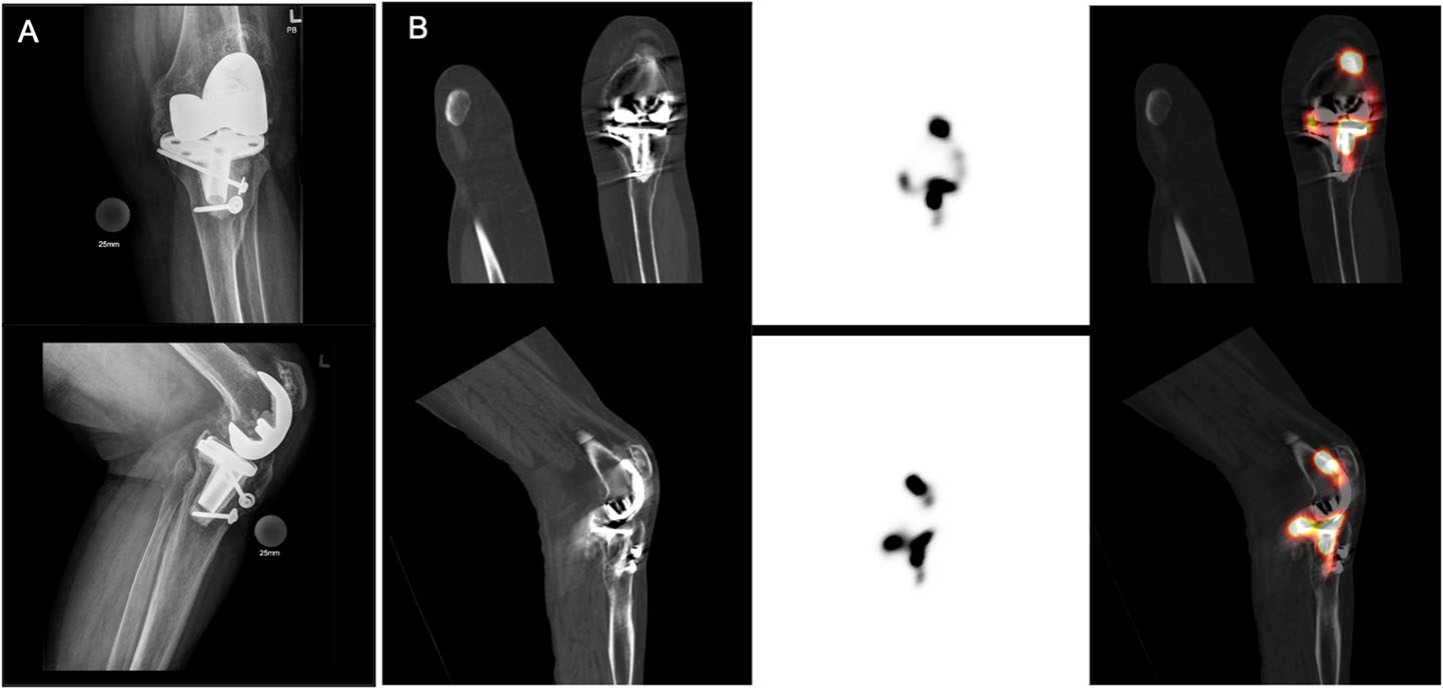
Tibial component loosening of a left knee total arthroplasty. The radiograph images demonstrate no evidence of loosening (first column). Coronal (top) and sagittal CT (second column), SPECT (third column), and fused SPECT/CT (fourth column) images showing abnormal tracer activity along the bone-prosthetic interface of the tibial component
